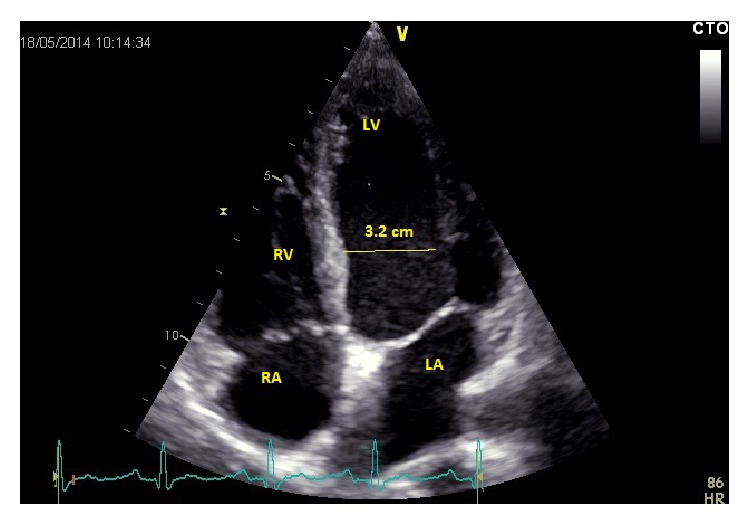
Transthoracic two-dimensional echocardiography showing normal sized ventricular cavities (LV dimension in systole is 3.2 cm) and normal heart valves. LV: left ventricle, LA: left atrium, RA: right atrium, and RV: right ventricle.
